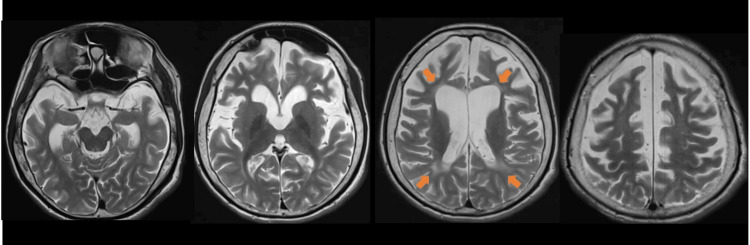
Brain magnetic resonance imaging at the age of 17 years before administration of the ketogenic diet. On axial T2-weighted images, diffuse cerebral atrophy is observed. Diffuse T2 hyperintense white matter lesion is also noted (arrows).
